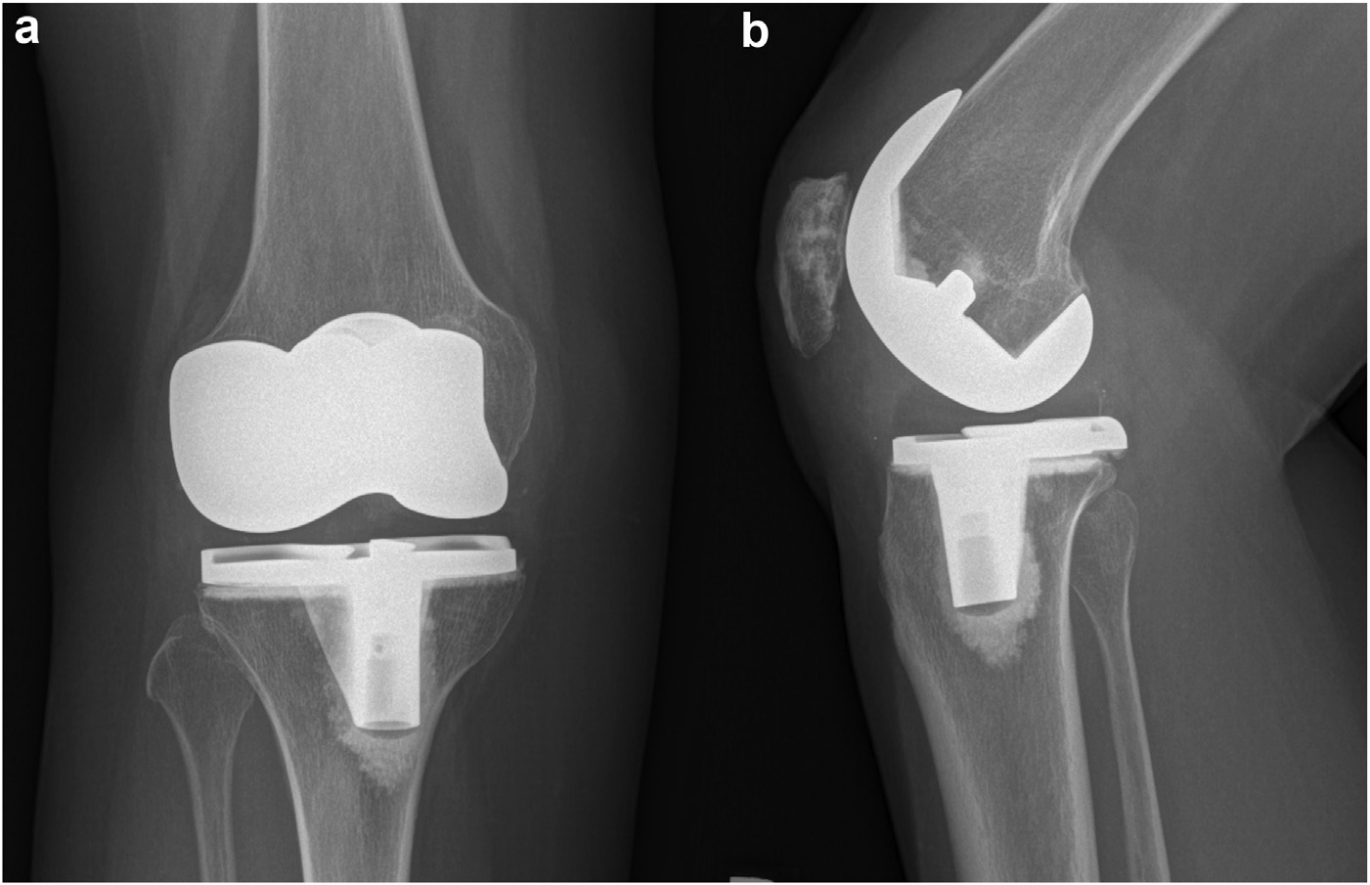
(a) Anterior-posterior and (b) lateral X-rays of the knee after total knee arthroplasty.
